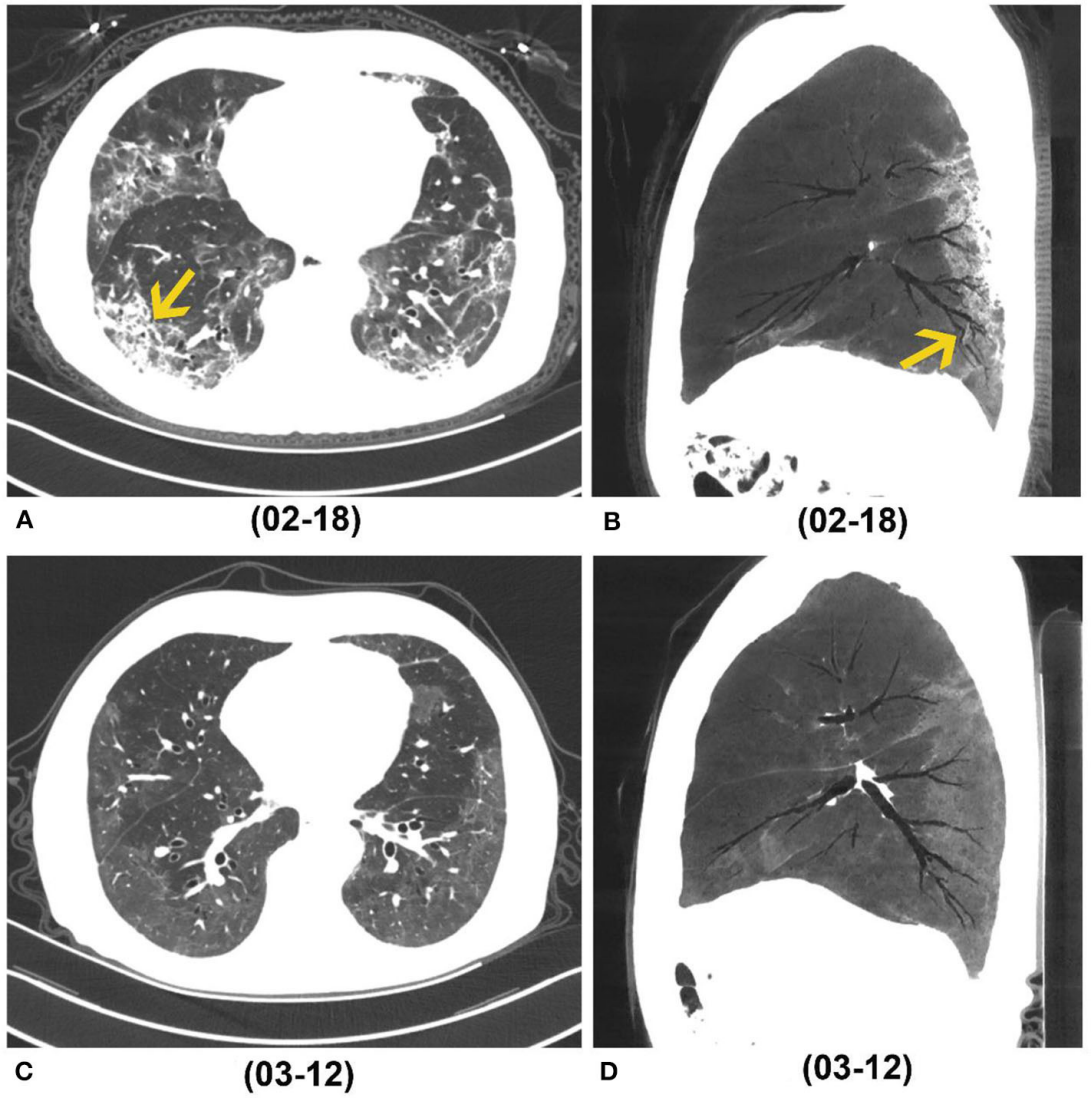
A 68-year-old man who caught a fever and cough on January 17, 2020. (A,B) Axial and sagittal CT (Min IP) performed on February 18 (peak CT, 32 days after symptoms onset) showed GGO, reticulation (yellow arrow in A) and bronchial distortion (yellow arrow in B) in the bilateral lungs. (C,D) Axial and sagittal CT (Min IP) performed on March 12 (last follow-up CT, 55 days after symptoms onset) showed residual GGO, while previous reticulation and distortion disappeared. Min IP, minimum intensity projection; COVID-19, coronavirus disease 2019; GGO, ground-glass opacity.
